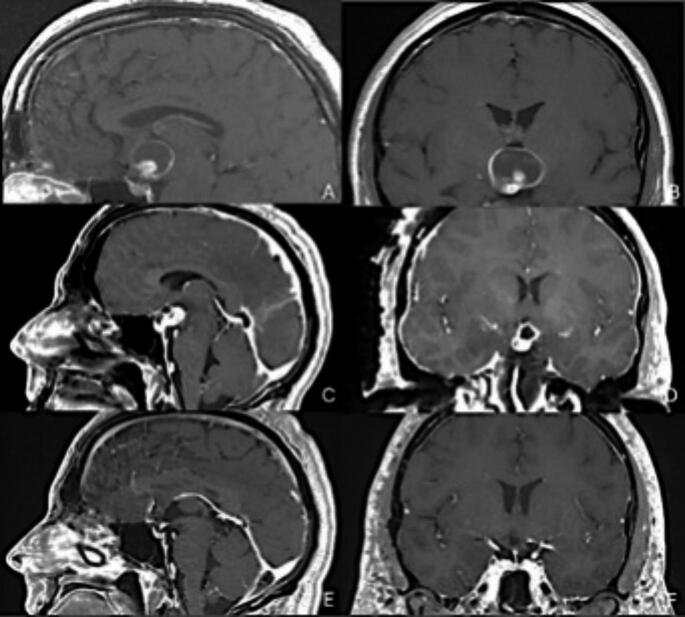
Panel A-B show preoperative MRI with a complex solid-cystic intraventricular lesion without calcification. Panel C-D show the immediate postoperative MRI demonstrating decompression of the cystic component of the tumor with residual enhancing tumor along the optic chiasm and hypothalamus following right sided cranioorbital craniotomy subfrontal approach for decompression and diagnosis. Panel E-F show an MRI at most recent follow up after treatment with combined BRAF/MEK inhibitor therapy, and ultimately discontinuation of both, with minimal residual enhancement
